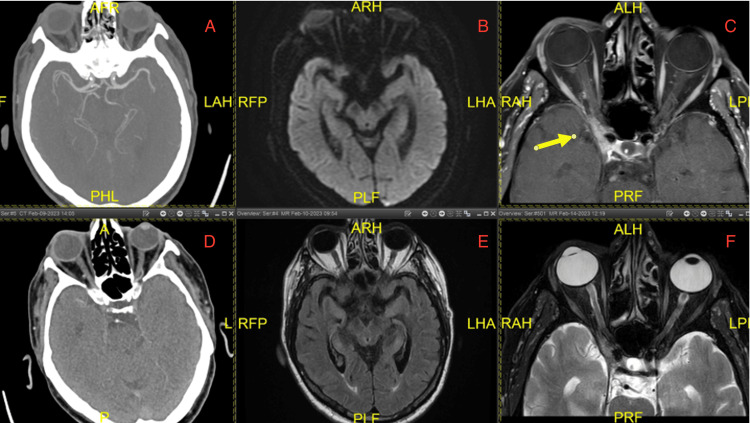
CTA and MRI of head and neck. (A) and (B): Computed tomography angiography (CTA) head and neck without any large vessel occlusion. (C) and (D): MRI brain with stroke protocol DWI and Flair without any evidence of stroke or metastasis. (E) and (F): T1 and T2 images of dedicated MRI orbits with contrast showing metastasis in the right orbit and cavernous sinus.
